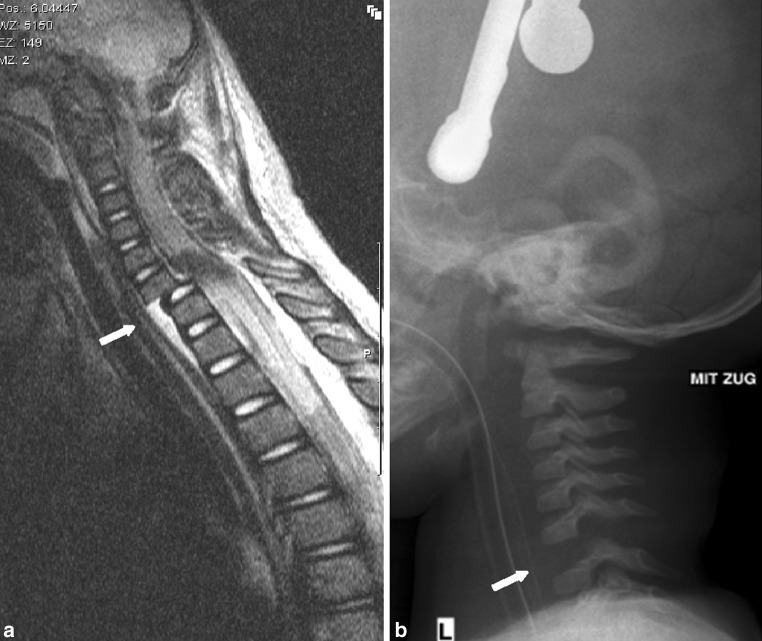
a T2 weighted MRI images after the spinal cord injury was detected clinically, showing a separation of the vertebral endplate from the vertebral body through the growth plate of C6 (typical for children) and the subsequent hematoma lifting the anterior longitudinal ligament off the vertebral body of C7 and Th1. There is a translation of C6 in relation to C7. Inhomogeneity of the signal in the spinal cord indicates severe spinal cord injury. Inhomogeneity of the signal at the interspinous ligament between C6 and C7 indicates an injury. b Radiograph under slight traction already showing a clear diastasis between vertebral body of C6 and C7 indicating complete disruption of the discoligamentous complex
